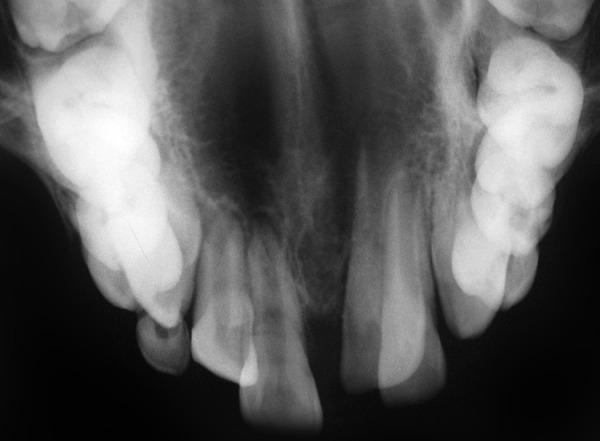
Six-month postoperative occlusal radiograph showing occlusal movement of left permanent maxillary central incisor
